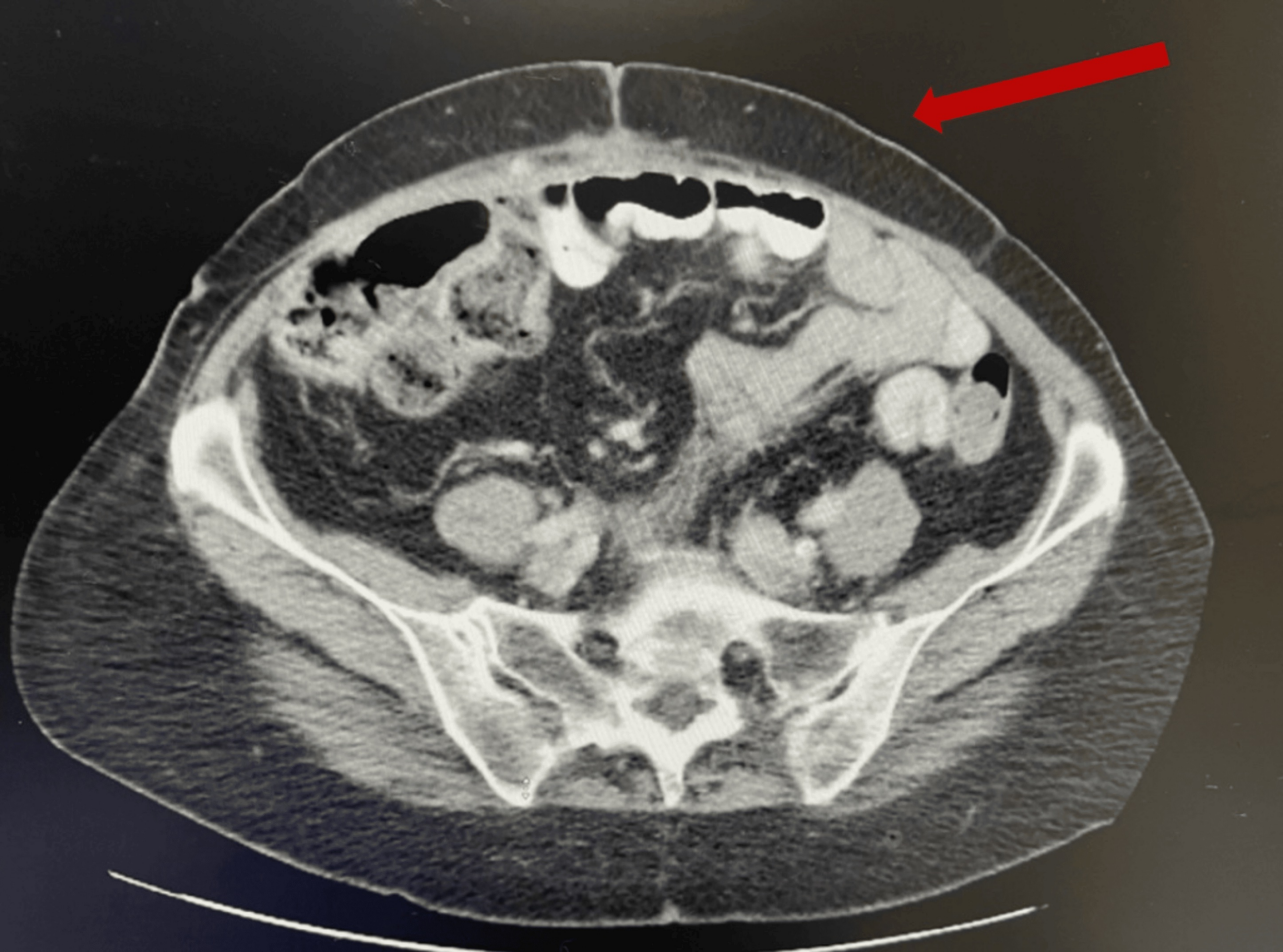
CT scan following retro-rectus component separation, transverse abdominis release, and mesh placement for reinforcement to repair the giant ventral hernia Repaired ventral hernia (red arrow) CT: computed tomography
